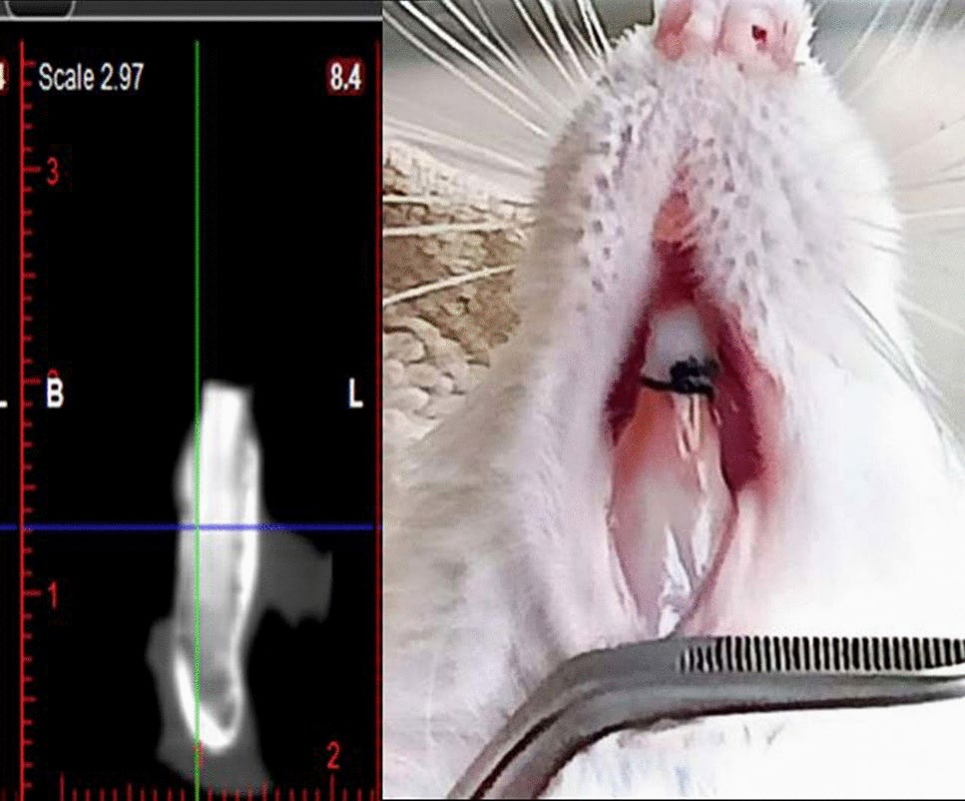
Clinical and radiographic photo of established periodontitis.
